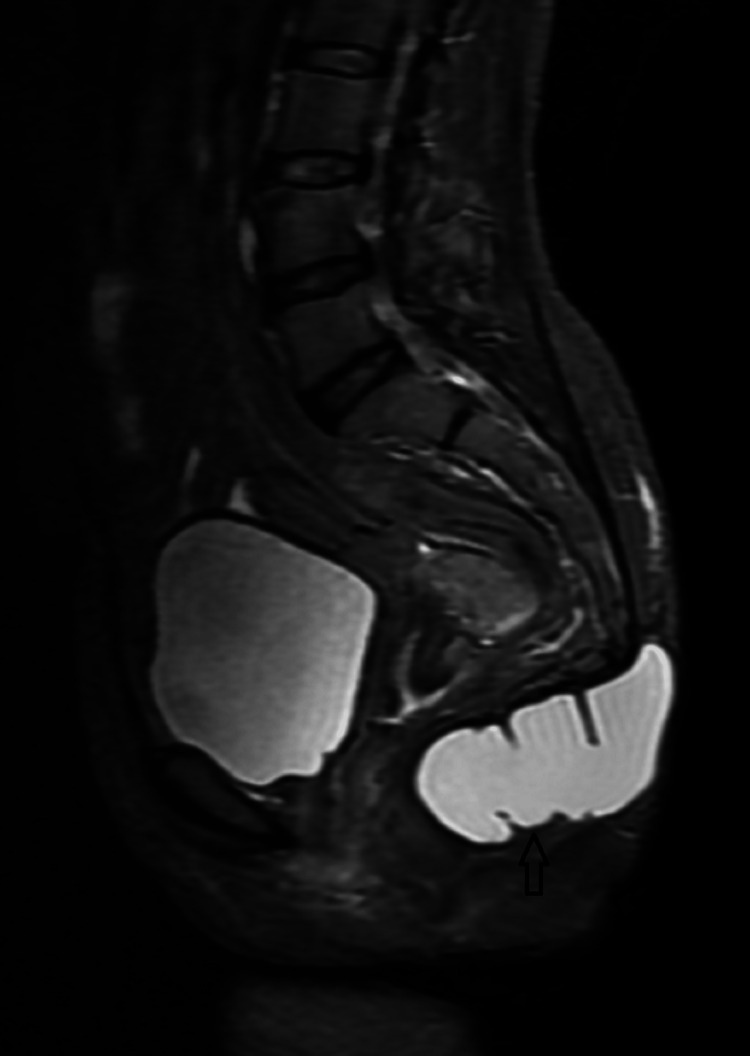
The sagittal section MRI STIR image shows that the lesion is appearing hyperintense (black arrow). STIR: Short tau inversion recovery https://assets.cureus.com/uploads/figure/file/450602/lightbox_27ec4db0313c11eda3a99f5a5f32899d-E3.png
